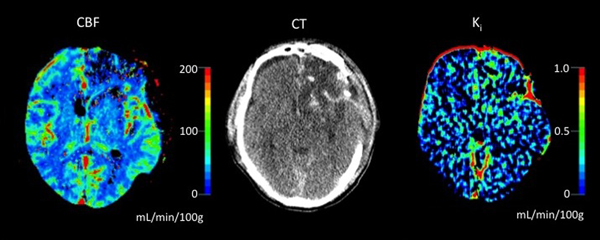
CBF (left) and permeability (right) maps, and contrast-enhanced CT scan (middle).
